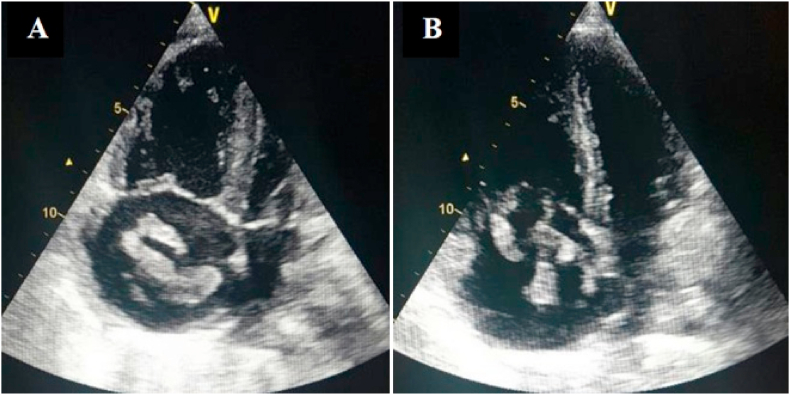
Thransthoracic echocardiography apical view showing a mobile thrombus inside the right atrium (A), protruding to the right ventriculaire in diastole (B).
